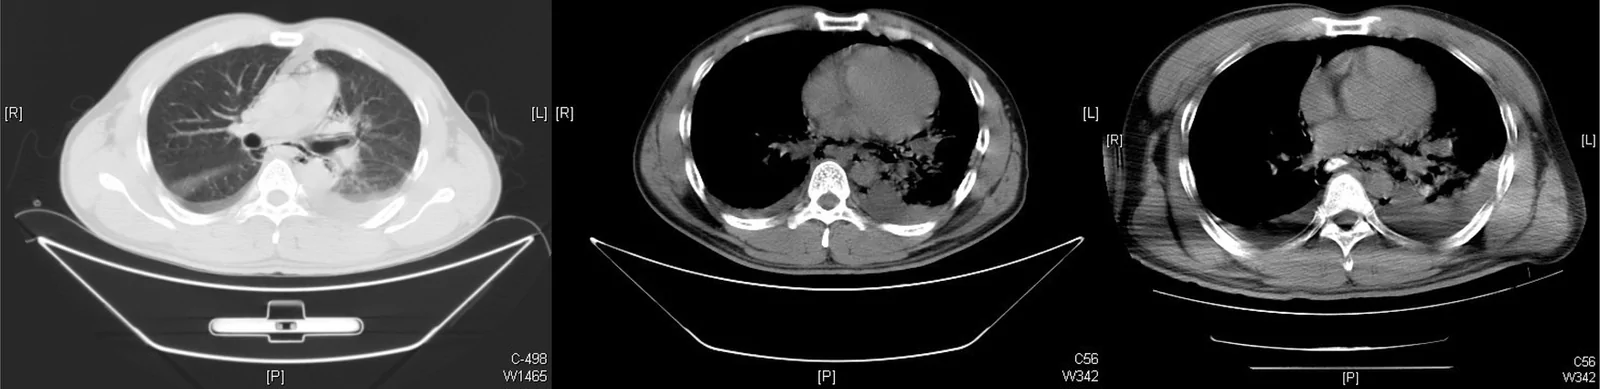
Chest CT on admission showing extensive pneumomediastinum and pleural effusion.
